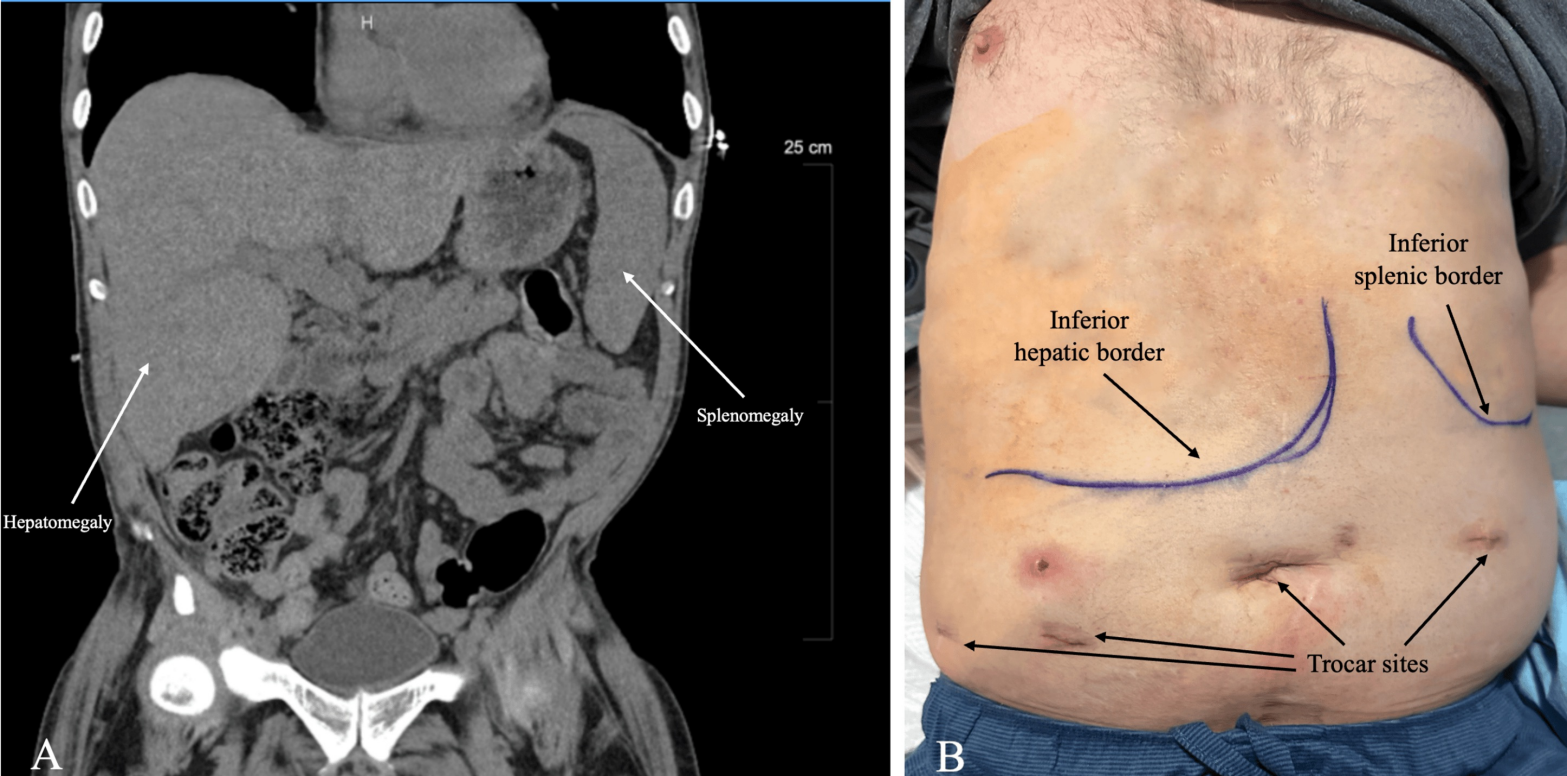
CT-guided preoperative organ marking for safe trocar placement. 3A) Coronal CT of the abdomen and pelvis used to delineate lower organ borders. 3B) Organ borders marked preoperatively using CT from 3A as a guide.
